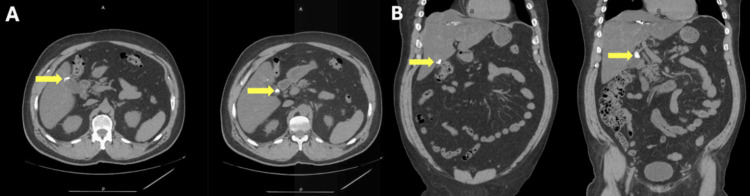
Axial (A) and coronal (B) CT of the abdomen Hyperdense masses in an apparently full gallbladder fossa (yellow arrows) with local inflammatory changes. Of note, the additional hyperdense mass in the right lobe of the patient’s liver was consistent with a retained foreign body resulting from penetrating trauma years prior. CT: computed tomography
